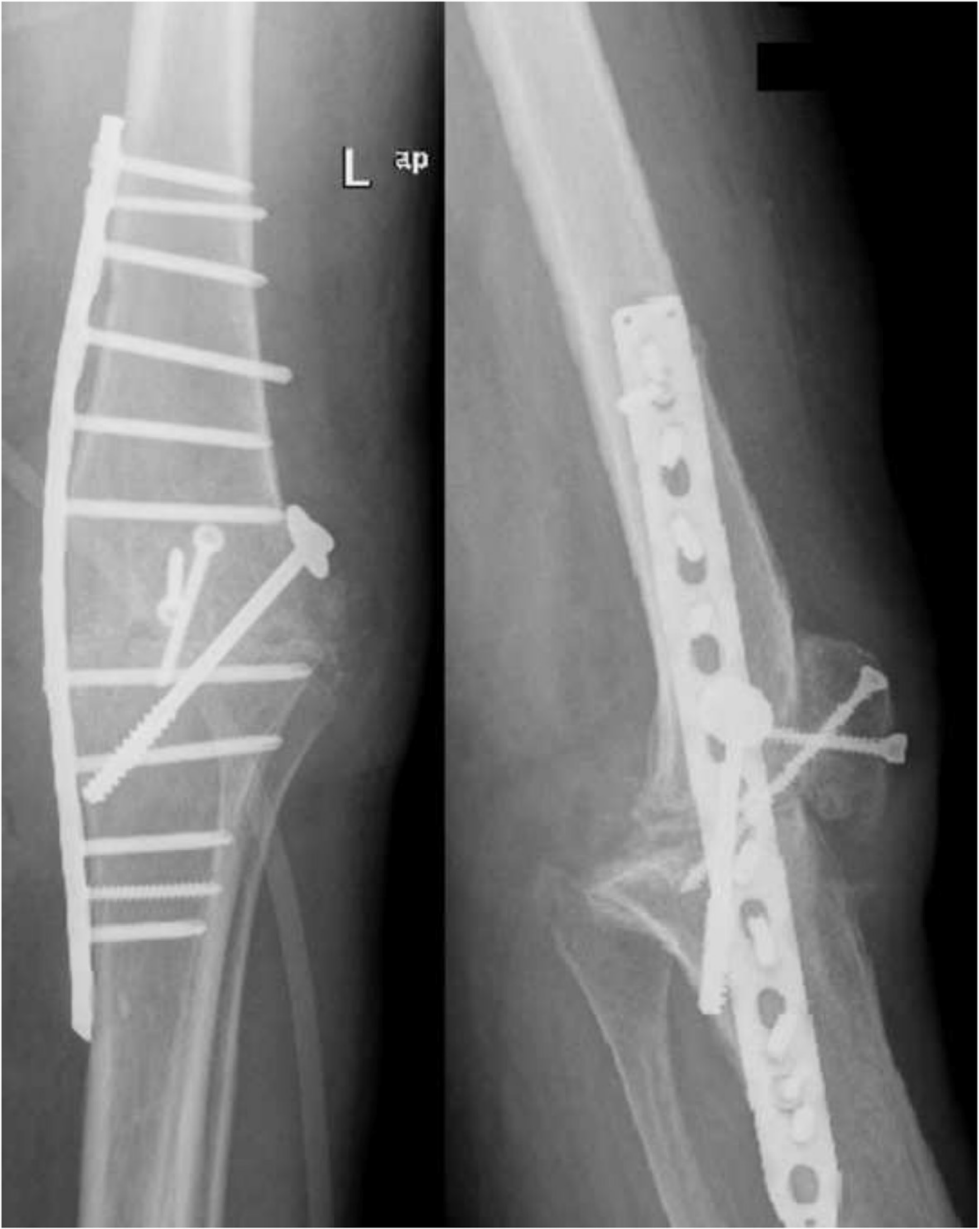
X-rays (AP and lateral view) after removal of Spacer and arthrodesis of left knee joint
